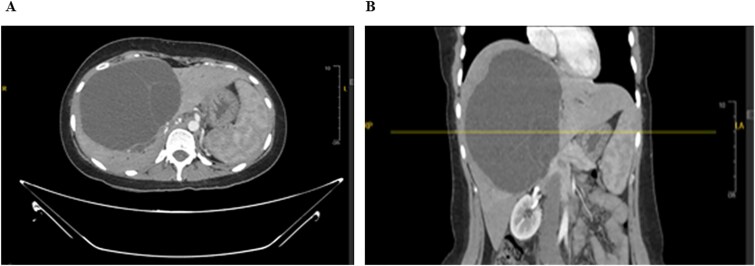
CT scan of the abdomen: Demonstrating the hepatic mass measuring involving the segments 4A, 4B, 5, 6, and 8. A) Transverse view, B) longitudinal view.
